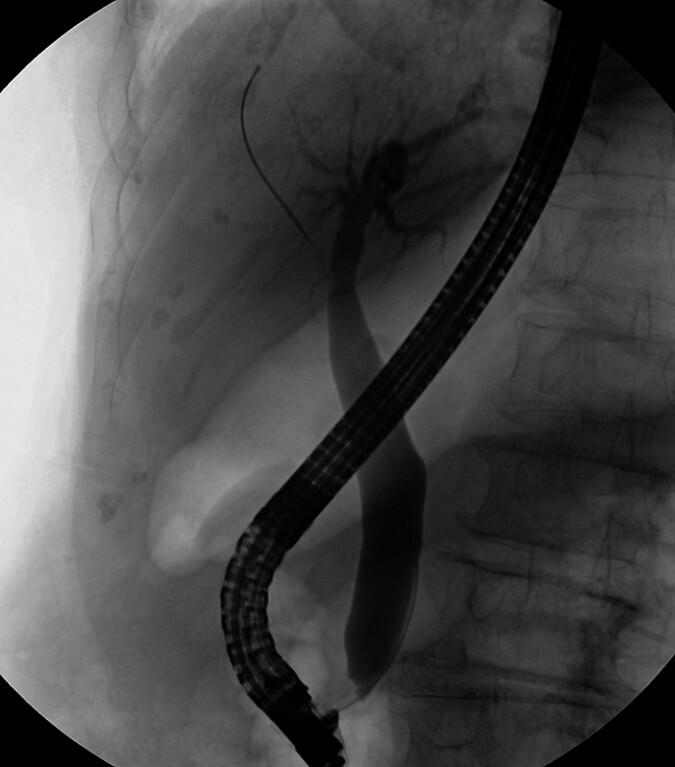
An X-ray image taken during ERCP. Dilation of the common bile duct was observed. ERCP, endoscopic retrograde cholangiopancreatography.
